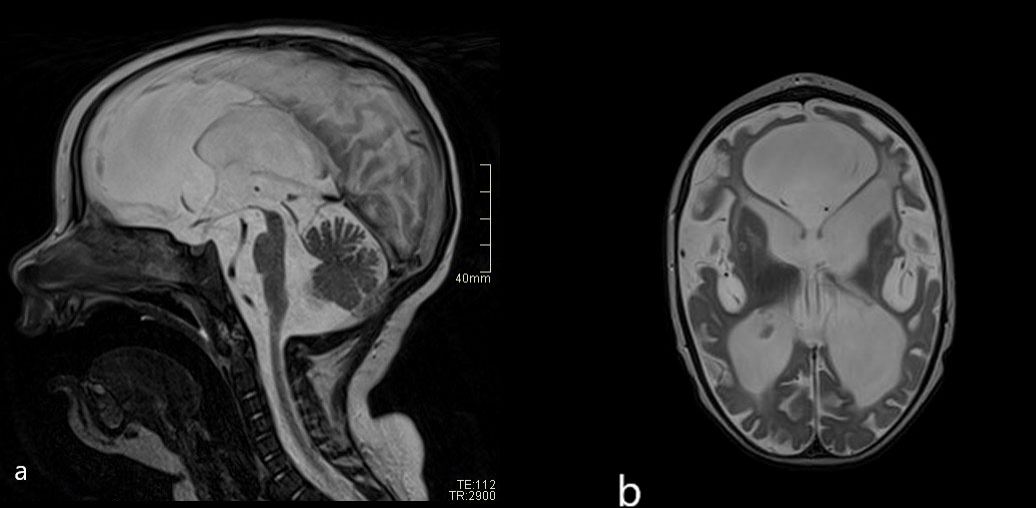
(A) Follow-up brain MRI scans (T2 sagittal and axial plane) after 1 year: severe atrophy of midbrain compared with initial MRI. (B) Severe reduction of brain volume and abnormal signal of the white matter—leucoencephalopathy.
